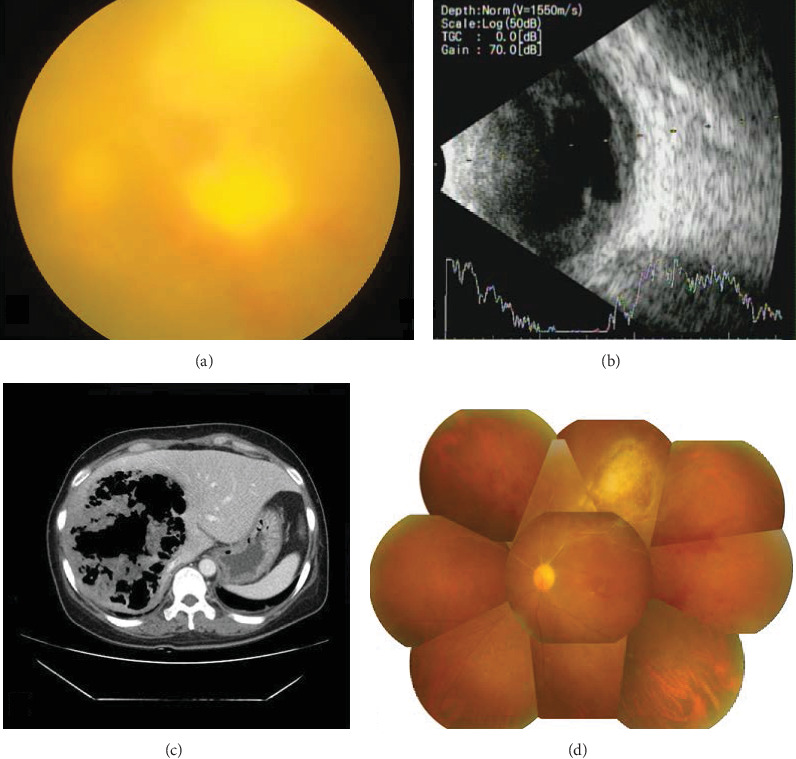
(a) Funduscopy shows a large necrotic lesion in the macular and superotemporal area with vitreous opacity of the left eye. (b) Ultrasound shows opacity and a diffuse abscess formation. (c) Abdominal computed tomography shows a gas-forming pyogenic liver abscess of the right lobe. (d) Fundus after 1 month of surgery, showing an atrophic lesion in the superotemporal area, and retinal hemorrhage around the macula.
